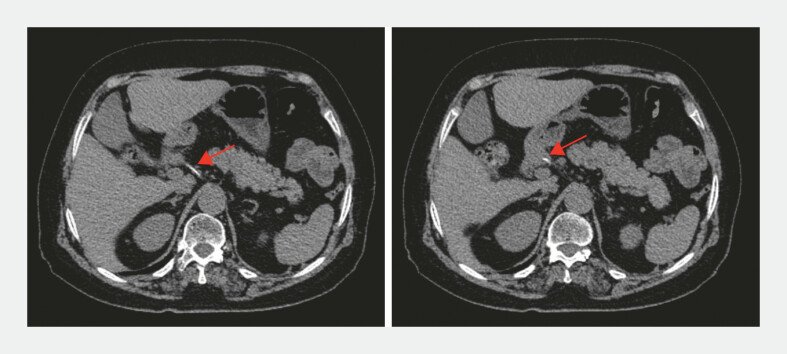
Computed tomographic scan showing a high-density shadow foreign body incarcerated in the duodenal bulb and abdominal cavity (red arrow).
